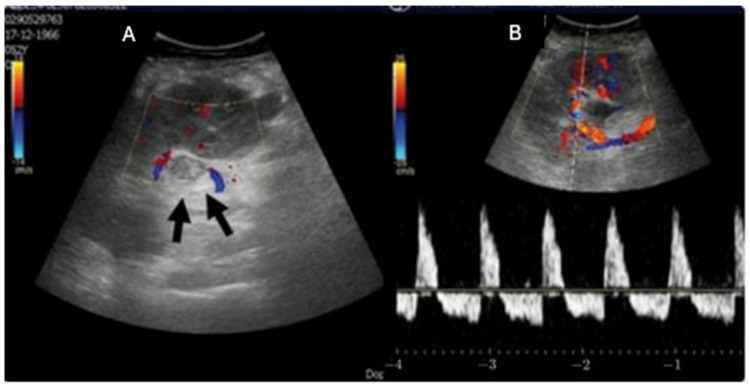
Thrombosis of renal vein demonstrated by color Doppler ultrasound. (A) Enlarged left renal vein with hypoechogenic content, without Doppler signal (black arrow). (B) Arterial reversal of diastolic flow. Image reused from “Unexpected success in early post-transplantation renal vein thrombosis: A case report and literature review”, by Santos JE et al., published by Clinical Nephrology Case Studies, accessed on 18 December 2025, under Public Domain (PDM 1.0) (https://creativecommons.org/publicdomain/mark/1.0/) [31]. Minor modifications were made to the title and descriptive captions. Labels (A,B) are new to this figure. Numbers on the scale were modified, but interpretation is unchanged.
